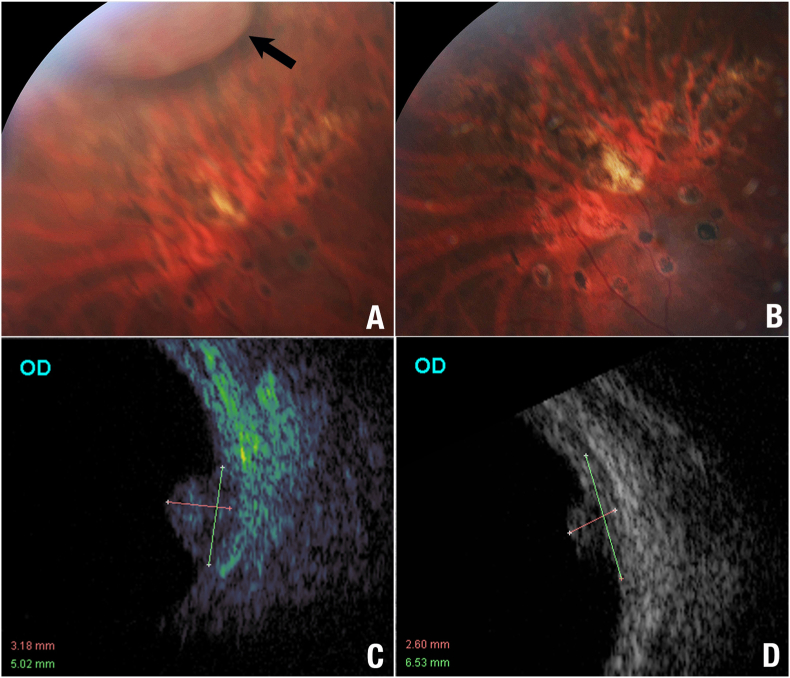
Case 2: Fundus photographs, A, note the retinal capillary hemangioma (black arrow) anterior to laser demarcation and prior to palladium-103 plaque therapy. B, the top right image reveals the same section 1-year after palladium-103 plaque brachytherapy. The tumor has regressed. C, an ultrasonographic B-scan image shows the retinal capillary hemangioma prior to irradiation. D, a B-scan shows the regressed tumor. The adjacent choroid thickening is likely due to intraretinal exudate or edema.
